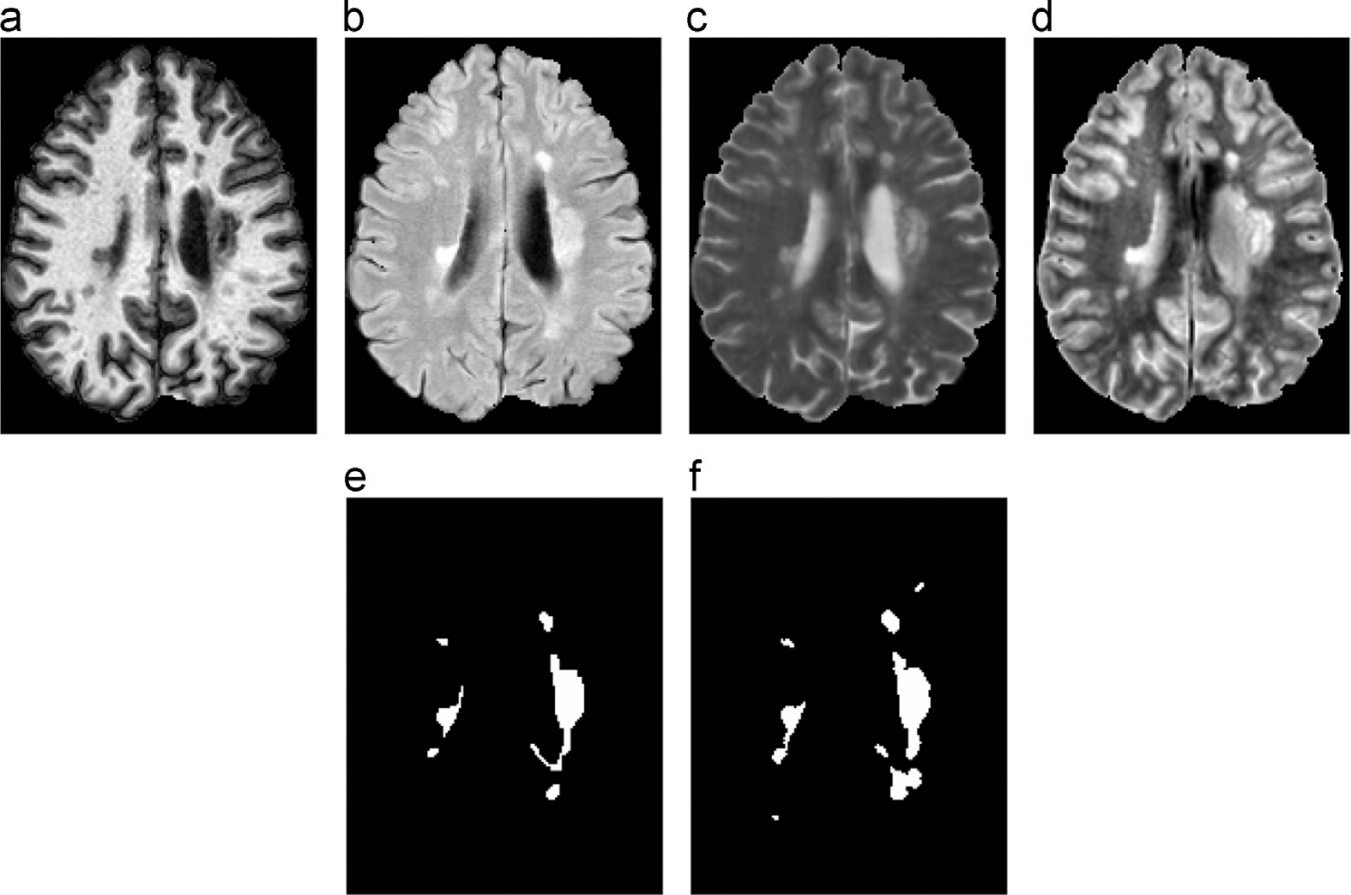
Shown are the preprocessed (a) MPRAGE, (b) FLAIR, (c) *T*_2_-w, and (d) PD-w images for a single time-point from one of the provided Training Set subjects. The corresponding manual delineations by our two raters are shown in (e) for Rater #1 and (f) for Rater #2.
